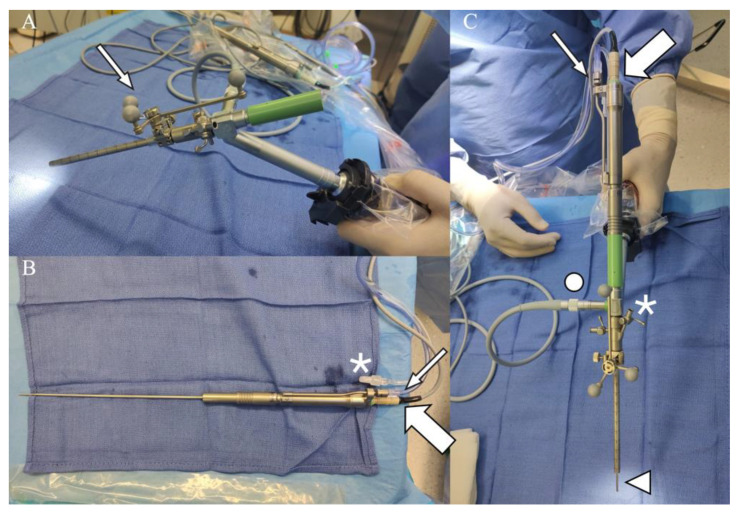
(A) 0° Gaab rigid endoscope (Karl Storz GmbH, Tuttlingen, Germany) with the attached three-point reference for navigation (arrow). (B) The NUA tool is presented (Endoscopic Neurosurgical Pen, Söring GmbH, Quickborn, Germany). The connection site of the aspiration (thin arrow) and ultrasonic oscillator (thick arrow) of the UA SYSTEM with the NUA are presented. The irrigation (asterisk) is connected directly to the endoscope. (C) Presenting the two instruments (NUA pen inserted into the GAAB endoscope) combined. The connections with the aspiration (thin arrow) and ultrasonic oscillating (thick arrow) system of the UA and the site (asterisk) where the irrigation is connected to the endoscope are presented. The NUA pen tip emerging through the endoscope (triangle), and the light source (circuit) and attached three-point reference for navigation are presented.
